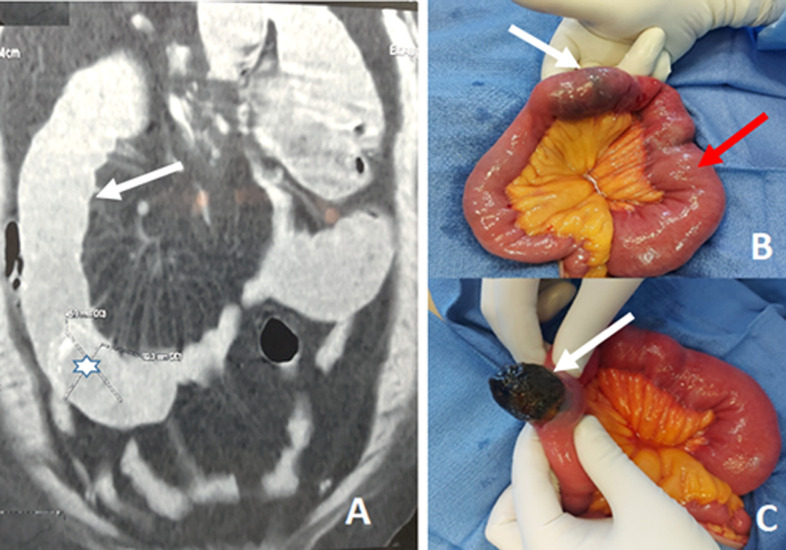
A) abdominal computed tomography scan (coronal section), showing dilated loops (arrow) of small bowel with a large gallstone with a calcified rim (star); B) per-operative view showing gallstone impacted in the ileus (white arrow) with dilated intestinal proximal loops (red arrow); C) per-operative view showing extraction of the gallstone through a longitudinal enterotomy (white arrow)
